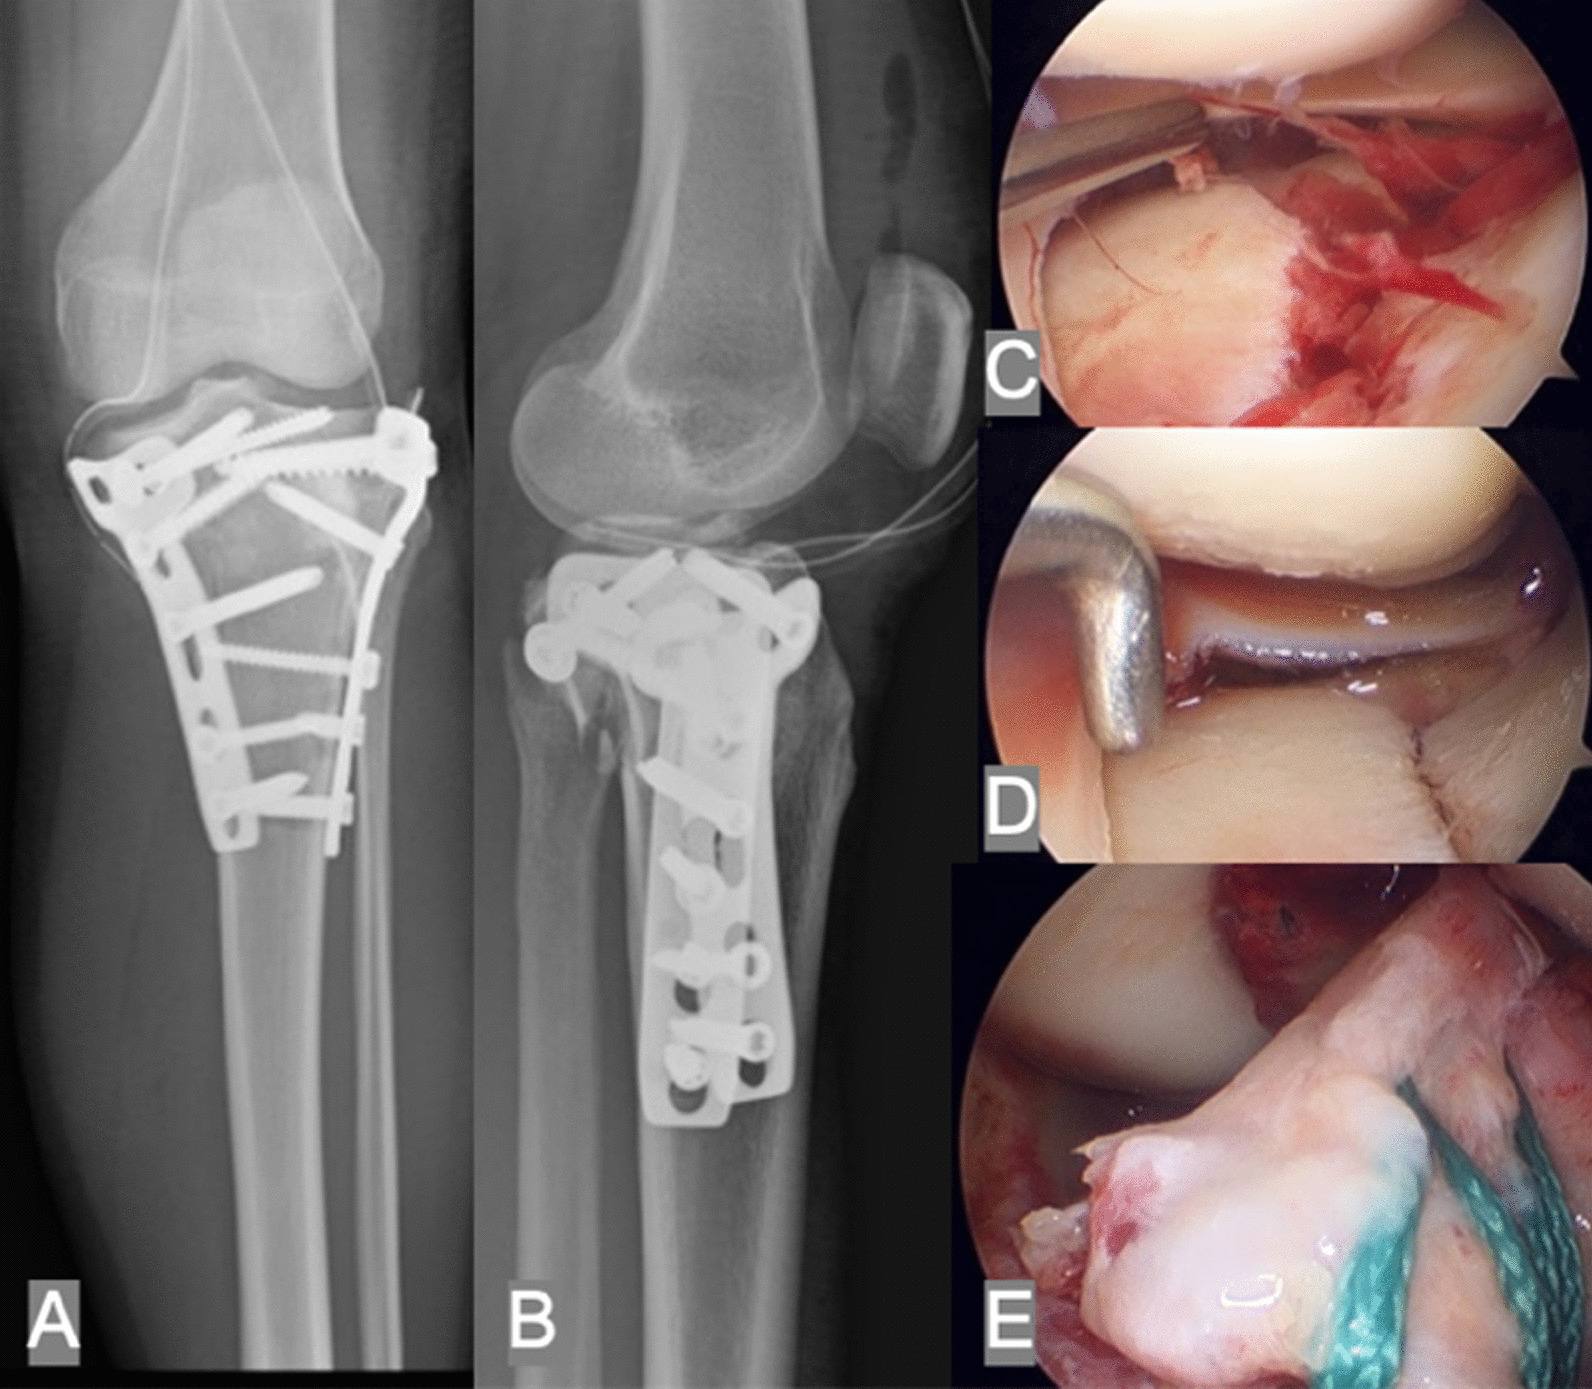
Arthroscopic-assisted reduction and internal fixation intraoperatively. A, B In addition to double plating, the posteromedial fragment was fixed with 2 interfragment screws. Both planes of radiographic alignment were well maintained. C, D, E Using the arthroscopic technique, joint depression due to the posteromedial fragment was directly visible and could be reduced perfectly through the fracture site. ACL avulsion was also treated simultaneously with arthroscopic suture fixation using 4 No. 5 Ethibond sutures
